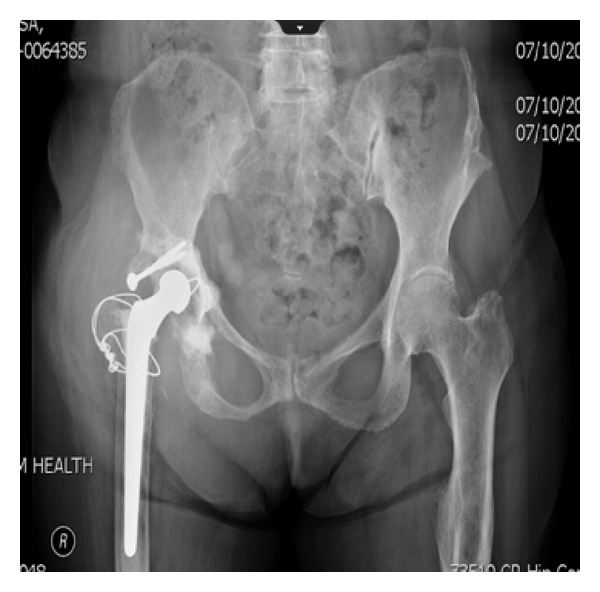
AP radiograph of pelvis revealing previous right total hip arthroplasty.
